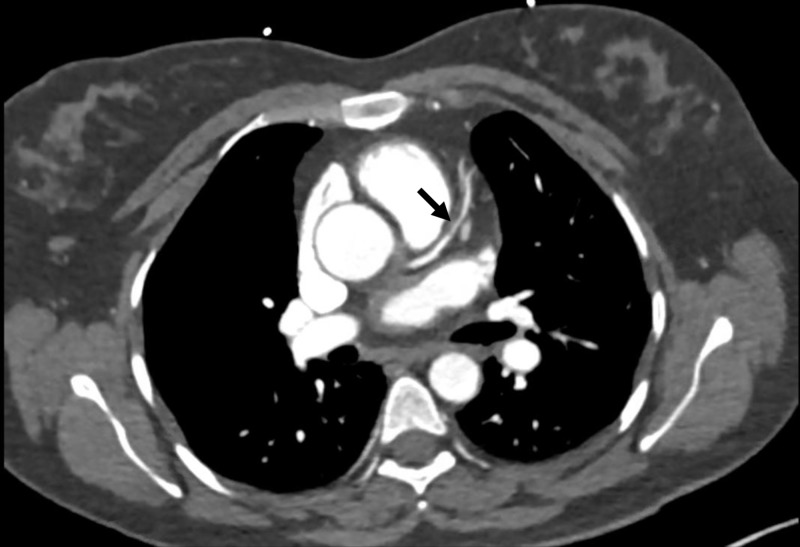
Coronary computed tomography angiography. Mild stenosis of the left anterior descending artery (arrow).
